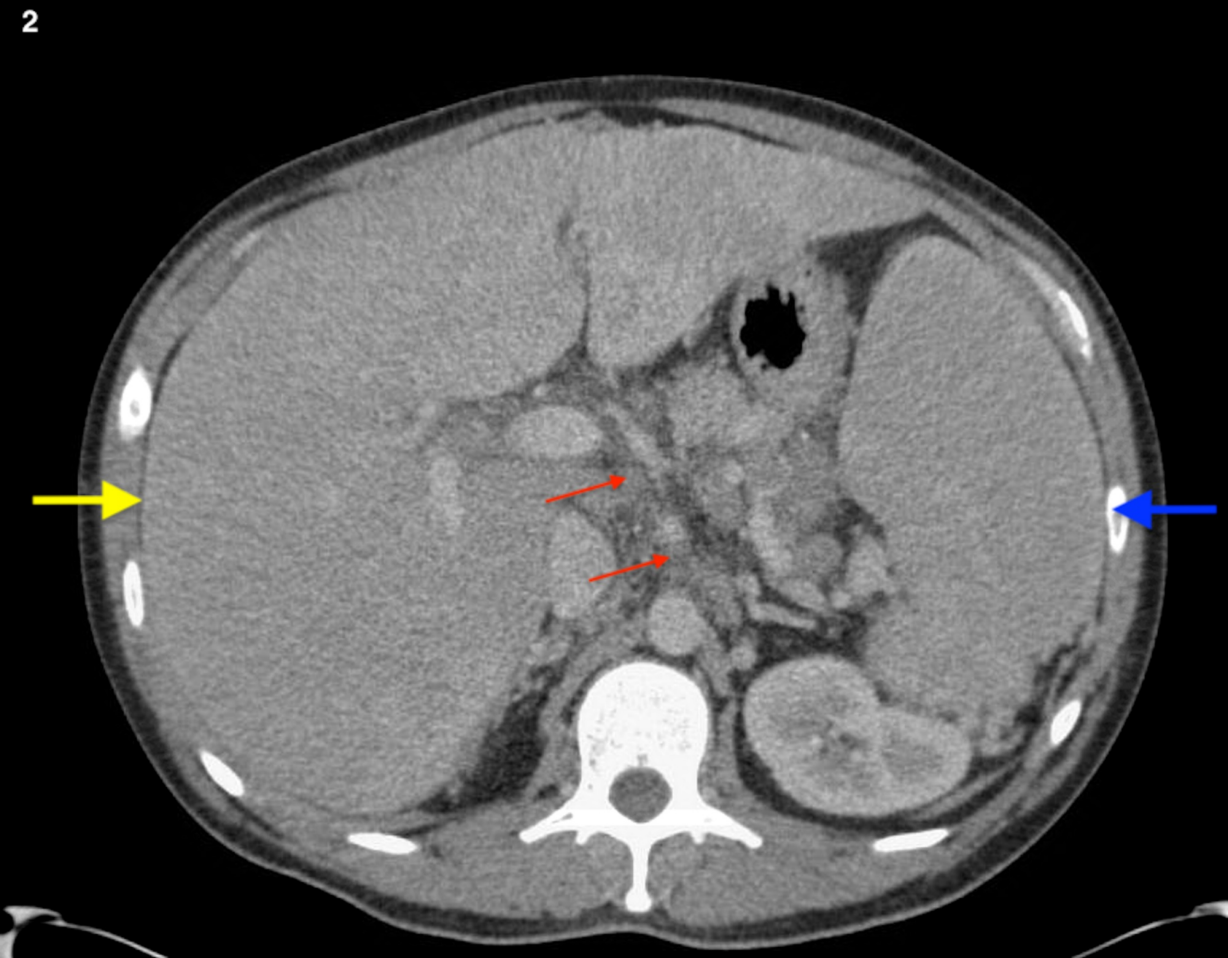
CT of the abdomen in a patient with hepatic sarcoidosis A massively enlarged liver (yellow arrow) and spleen (blue arrow) with peripancreatic and periportal lymphadenopathy (red arrow)
